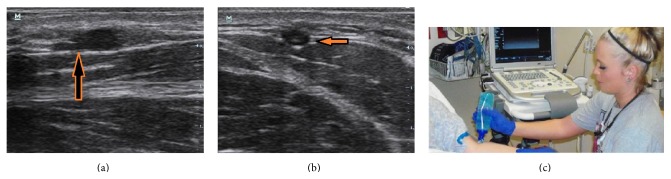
(a) Catheter tip (hyperechoic or white) shown accessing a vein (hypoechoic or black square) in the oblique access; the catheter is highlighted in by the orange arrow. (b) A catheter tip (hyperechoic or white) shown accessing the peripheral vein (hypoechoic or black circle) circle in the coronal plane delineated by the orange arrow. (c) A nurse preparing to perform US guided IV access notes the location of the ultrasound machine to allow for line of site of both the machine and the patient.
